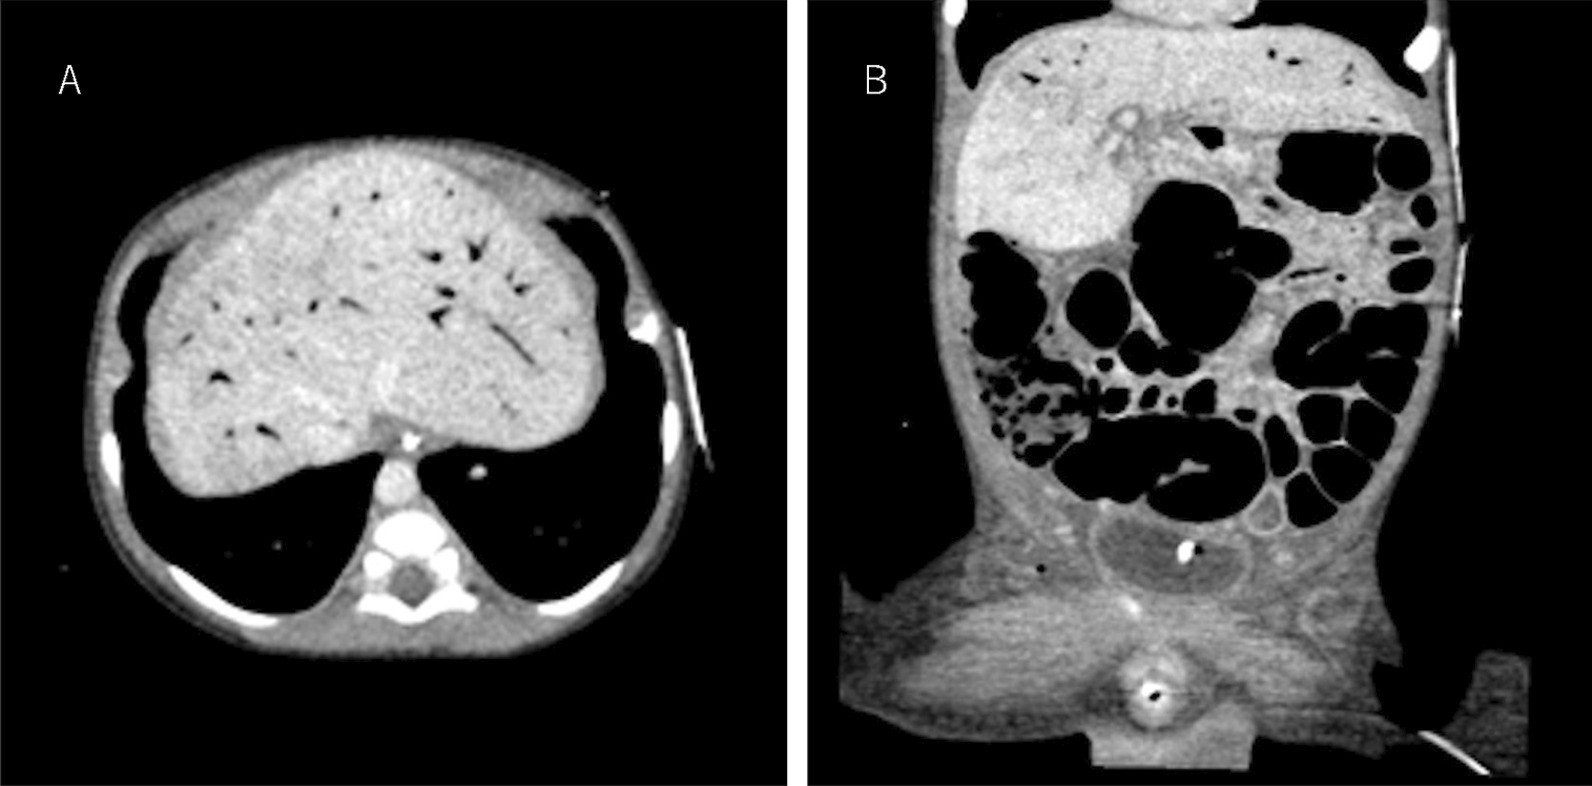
Contrast-enhanced computed tomography (CT) findings. A CT image depicting gas in the portal vein. B Extensive bowel dilatation and gas in the right colon wall without intra-abdominal free air are noted
